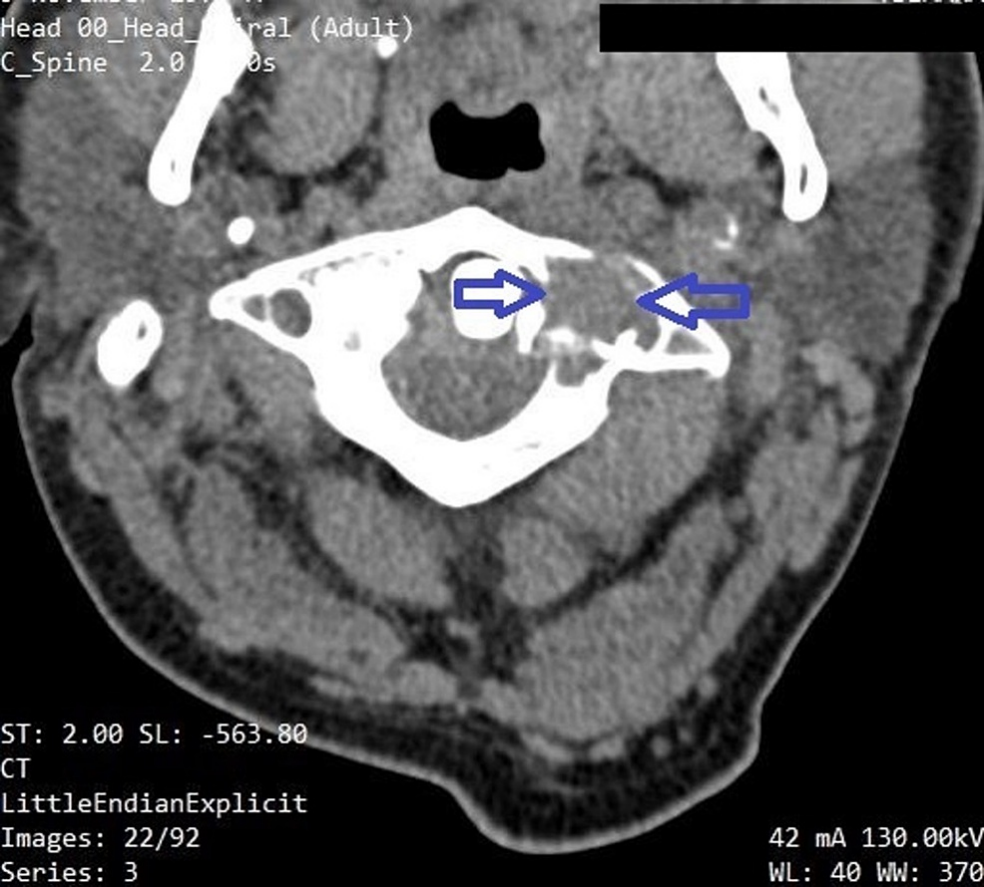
A CT scan showing an extensive osteolytic damage to the atlas (blue arrows).
